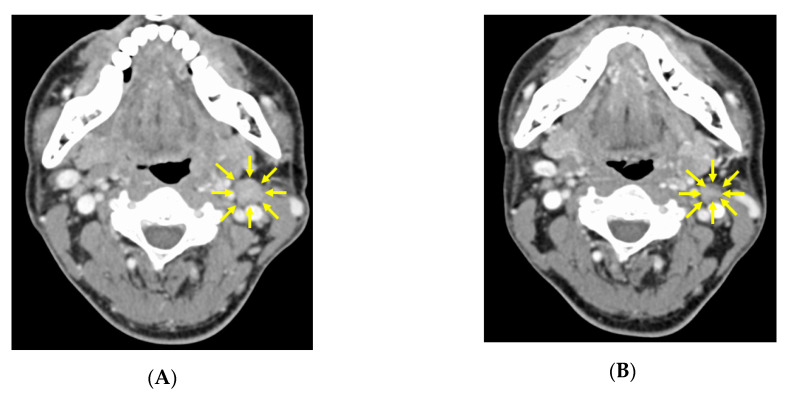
CT imaging findings of the left cervical lymph node region before and after neoadjuvant chemotherapy. (A) Before neoadjuvant chemotherapy, a 12 × 13 mm swollen lymph node is evident in the left upper internal deep cervical lymph node region (arrows). (B) After the second neoadjuvant chemotherapy course, the enlarged lymph node has shrunk to 10 × 10 mm (arrows).
